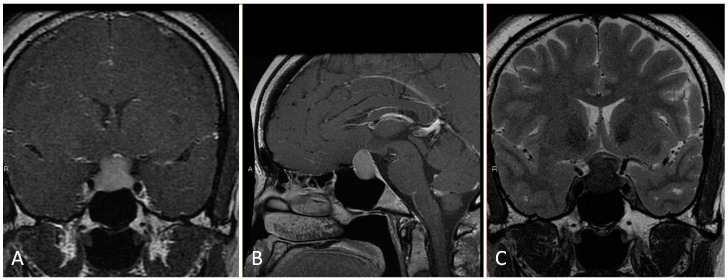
Preoperative imaging of a 38-year-old patient with bitemporal hemianopsia and a sellar lesion consistent with Rathke’s cleft cyst. (A) Coronal T1 post-contrast MRI. (B) Sagittal T1 post-contrast MRI. (C) Coronal T2 MRI.
